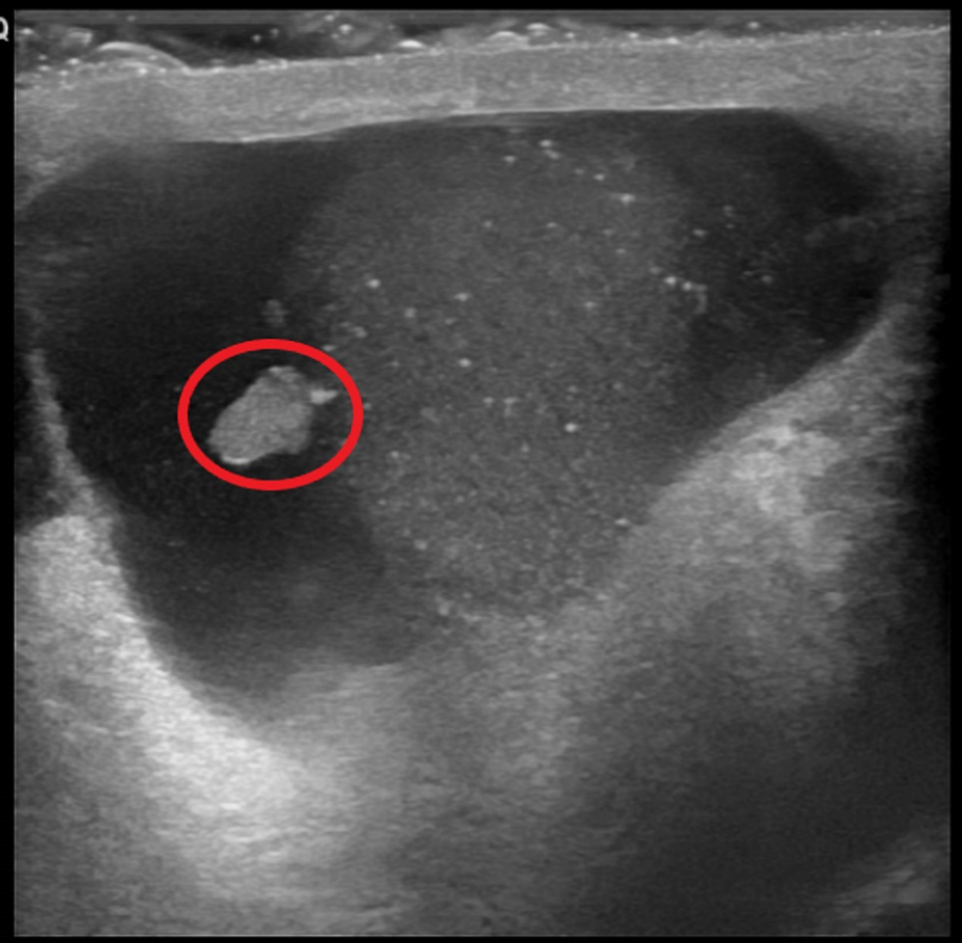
Ultrasound of the right testicle showing a hyperechoic mass on the inferior pole of the right testicle (red circle)
